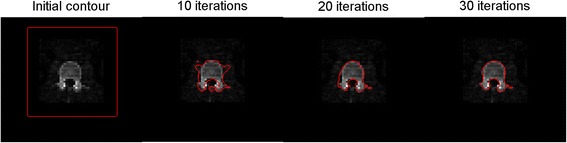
Example of the active contour evolution process from initial to final contour, depicting the detection of the outer bone contour of lumbar vertebrae in a single CT slice
